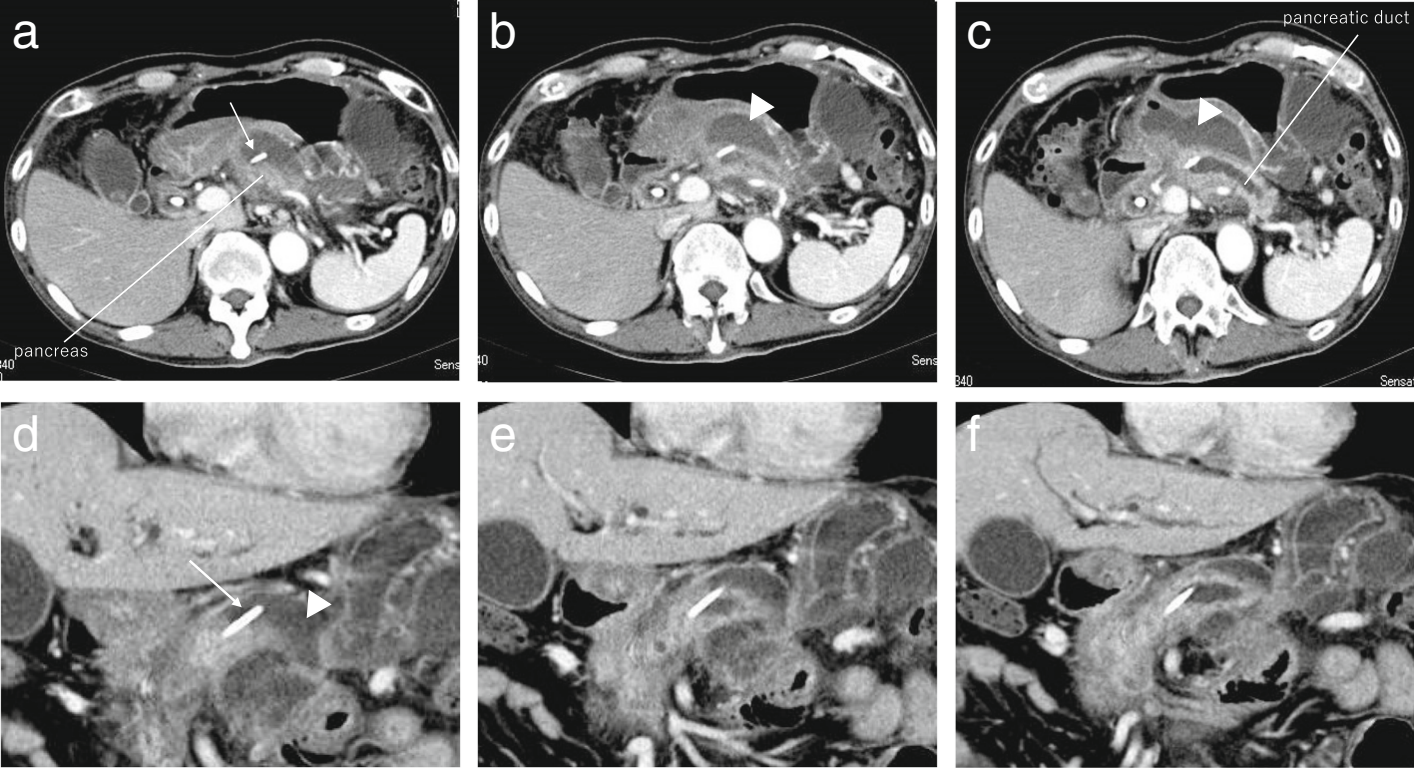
Enhanced computed tomography of the abdomen. A pancreatic stent protruded from the pancreatic body (arrow), and there was fluid collection around the greater omentum (arrowhead). a–c Axial view. d–f Coronal view
